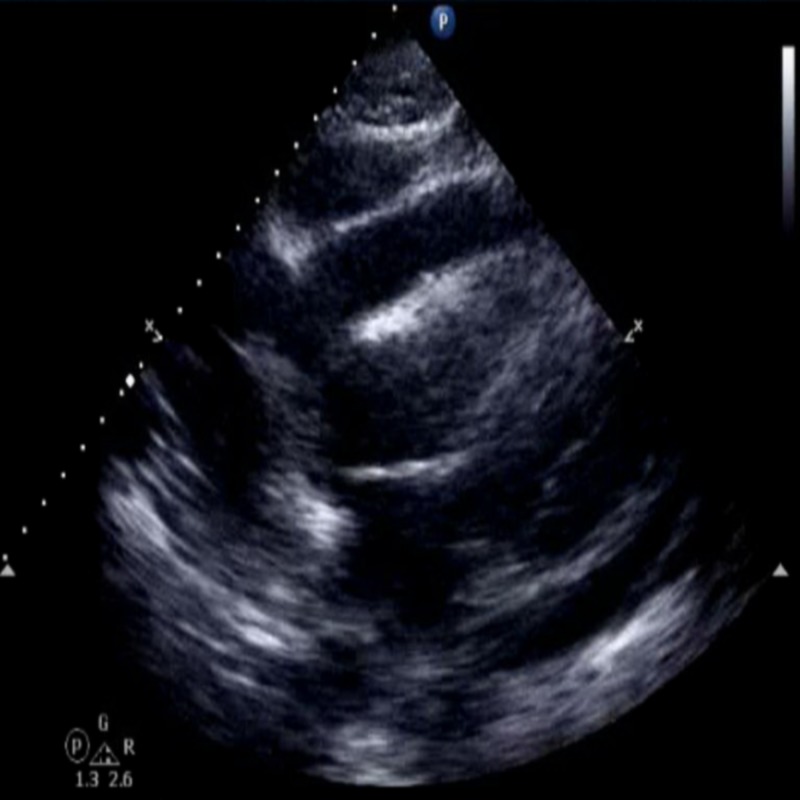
Echocardiogram; subscostal view. Revealing a moderate to large circumferential pericardial effusion surrounding the left ventricular (x).
